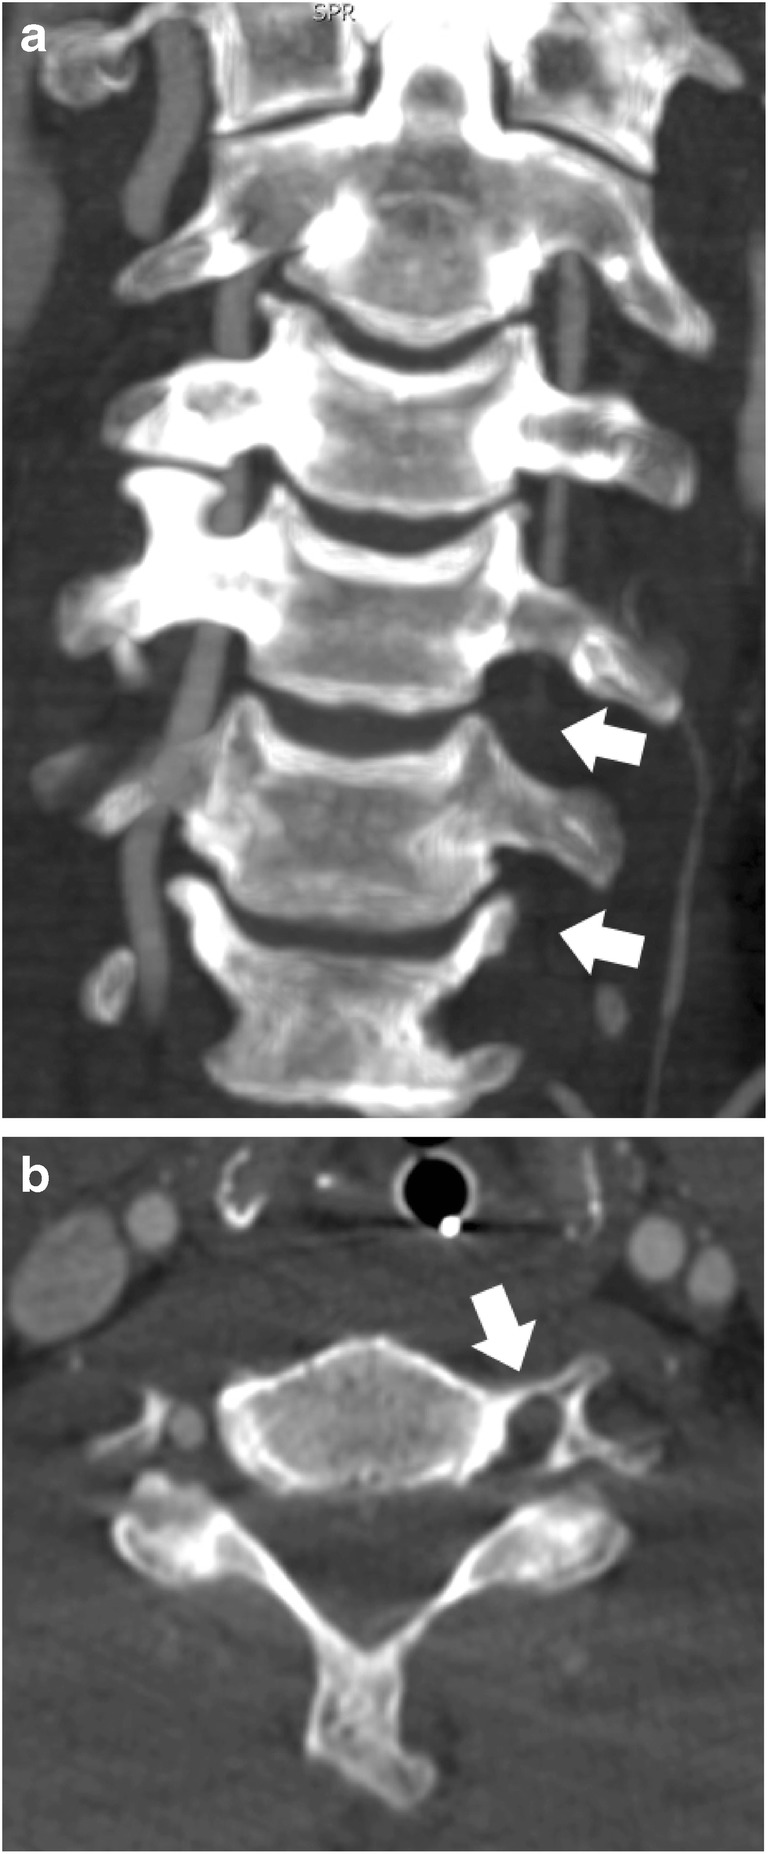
Grade IV BCVI of left VA on CTA in a 50-year-old male whose head hit the windshield in a MVA. a Coronal and b axial reformats show the absence of contrast in the lumen (arrows)
